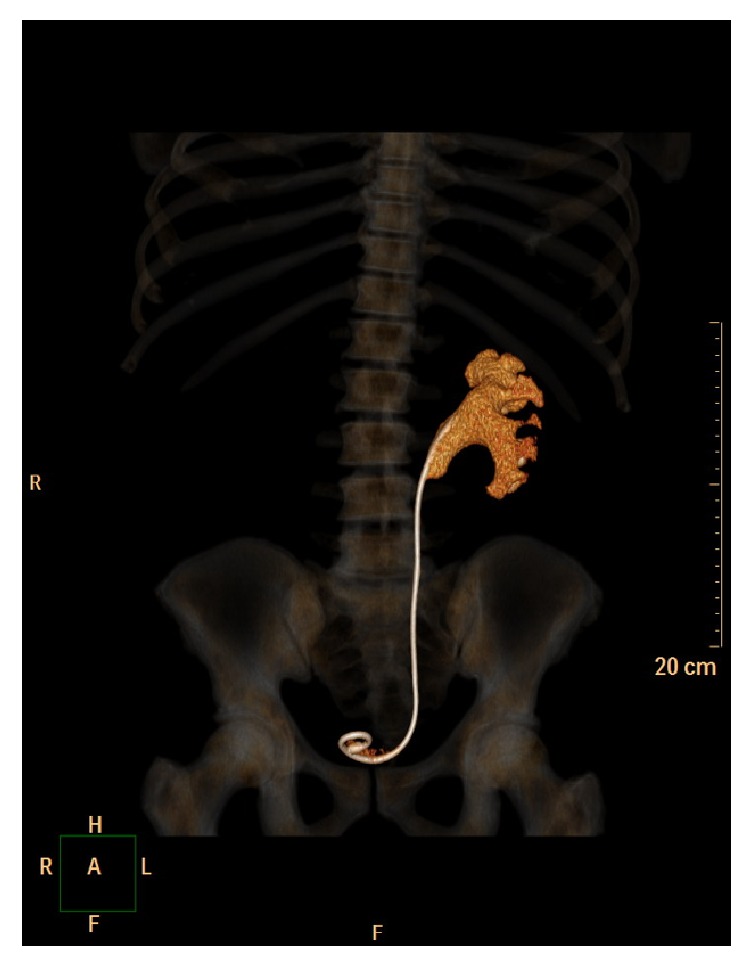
Abdominal computed tomography (coronal cut) showing complete coraliform calcification on double-J stent.
